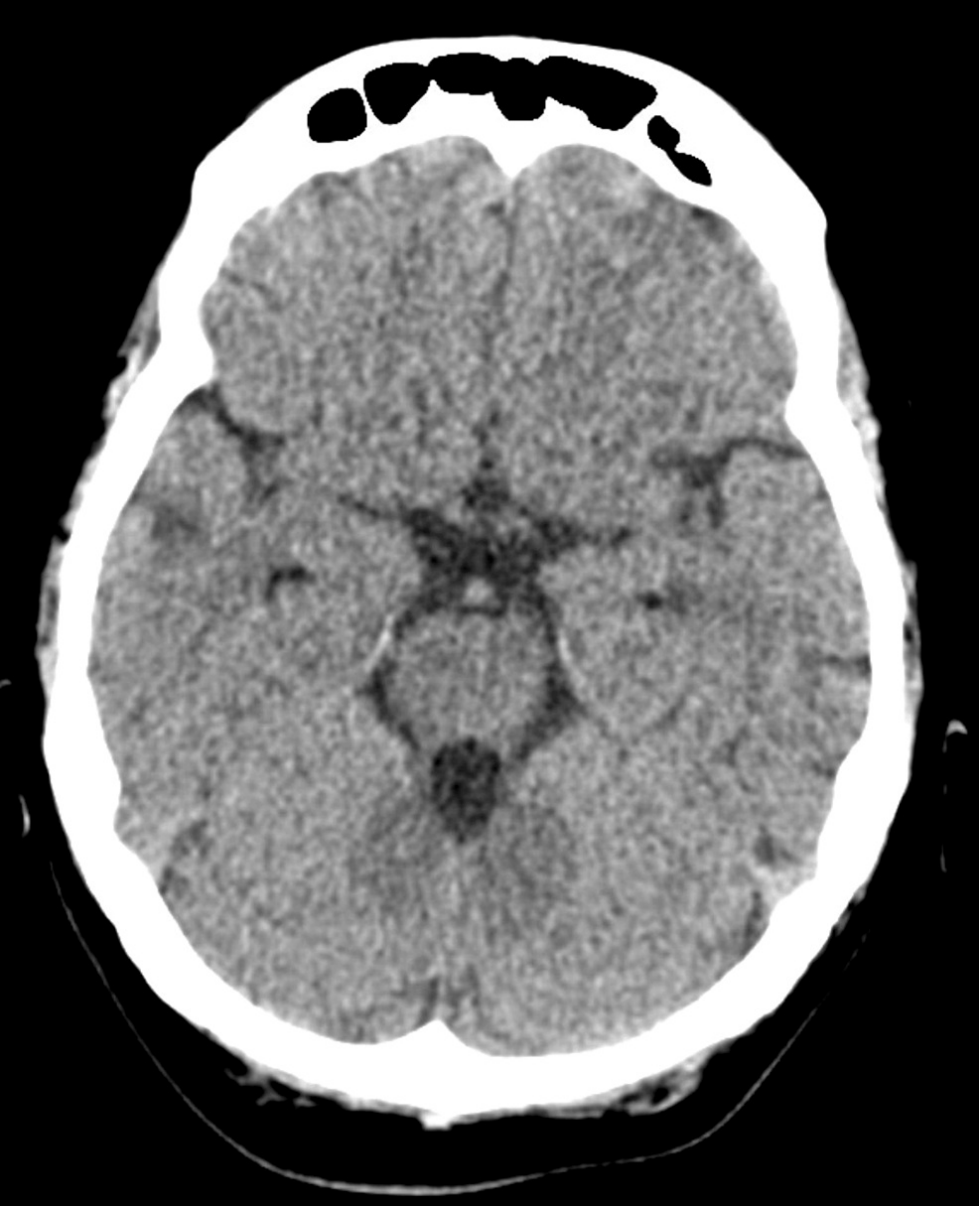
Vertical brain CT scan which revealed normal findings.
